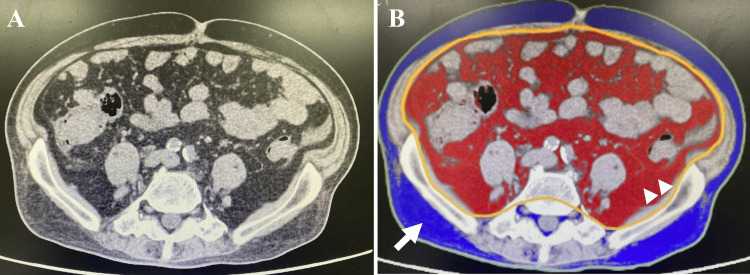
Abdominal CT slice at the level of the umbilicus, corresponding approximately to the L4 vertebral level, obtained upon admission. A: Plain CT scan at the level of the umbilicus. B: The visceral fat area was measured using a 320-row area detector CT scanner (CANON) with a slice thickness of 5 mm. The same plain CT scan at the level of the umbilicus, with visceral fat highlighted in red and subcutaneous fat highlighted in blue.The CT scan taken at the level of the umbilicus upon admission revealed a visceral fat area of 165 cm² (B, highlighted in red, indicated by arrow heads), indicating visceral fat accumulation. The subcutaneous fat area (B, highlighted in blue, indicated by arrow) was 109 cm².
